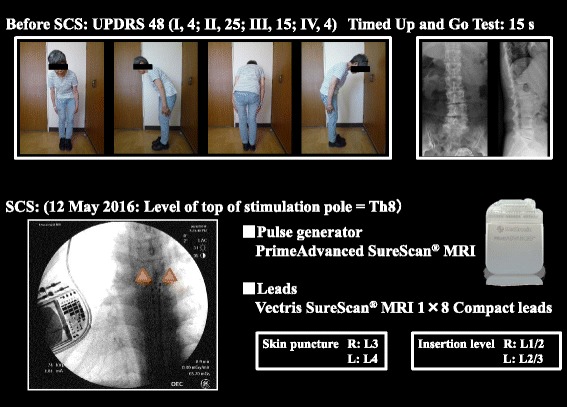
Painful camptocormia and SCS device. The patient’s camptocormia with Pisa syndrome to the right (top *left*). Spinal X-ray revealed mild degenerative lumbar spondylosis (top *right*). For SCS, a PrimeAdvanced SureScan^®^ MRI pulse generator was implanted and a pair of Vectris SureScan^®^ MRI 1 × 8 Compact leads were inserted percutaneously
